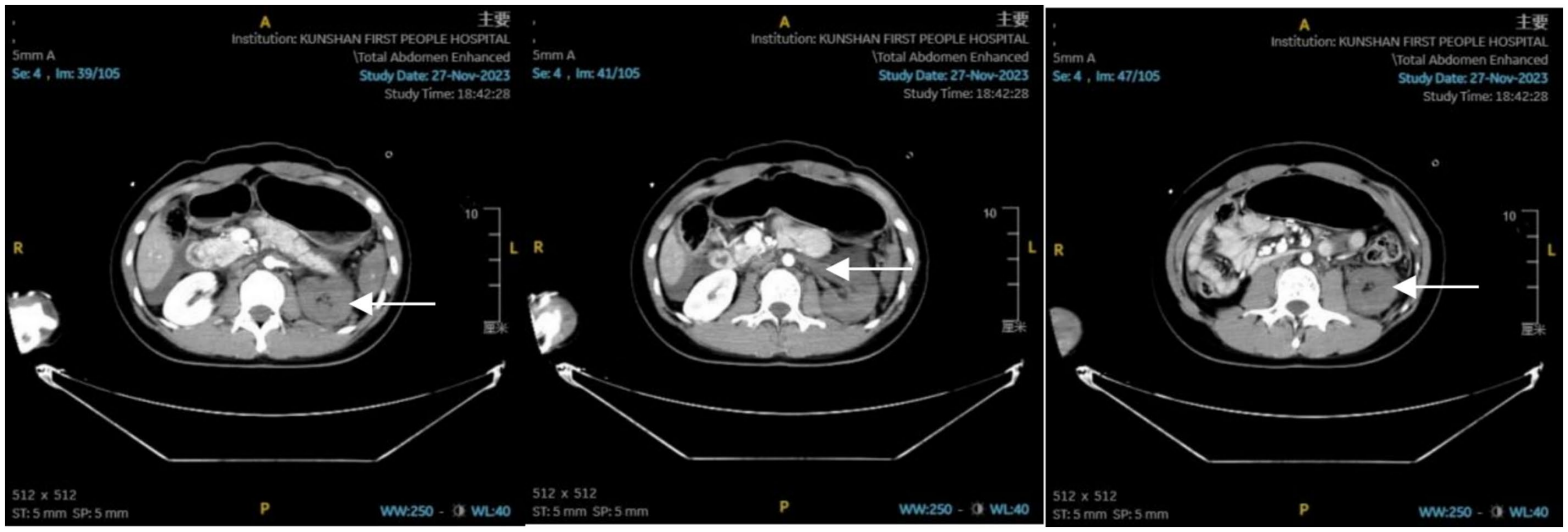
Abdominal enhanced CT report: contusion of the left hepatic lobe; contusion of the spleen; contusion of the left adrenal gland; embolism of the left renal artery; presence of abdominal and pelvic effusion.
